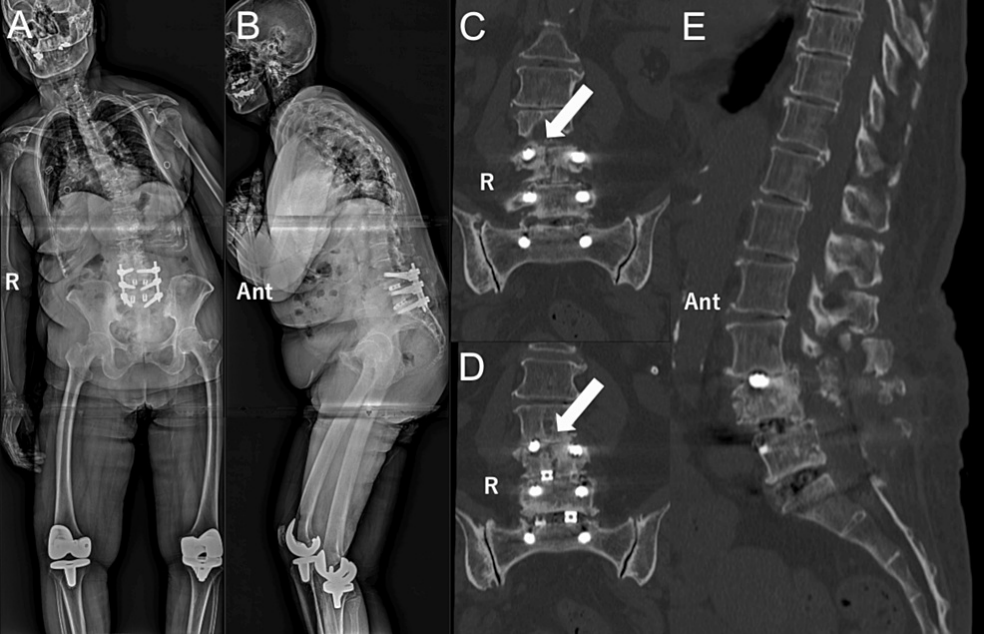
Radiographic images after the vertebral fracture of L4 A: anterior-posterior projection; B: lateral projection; C, D: coronal computed tomography images; E: sagittal computed tomography image. Because of the vertebral fracture of L4 (white arrows), the spinal deformity was aggravated (Ant: anterior, R: right).
